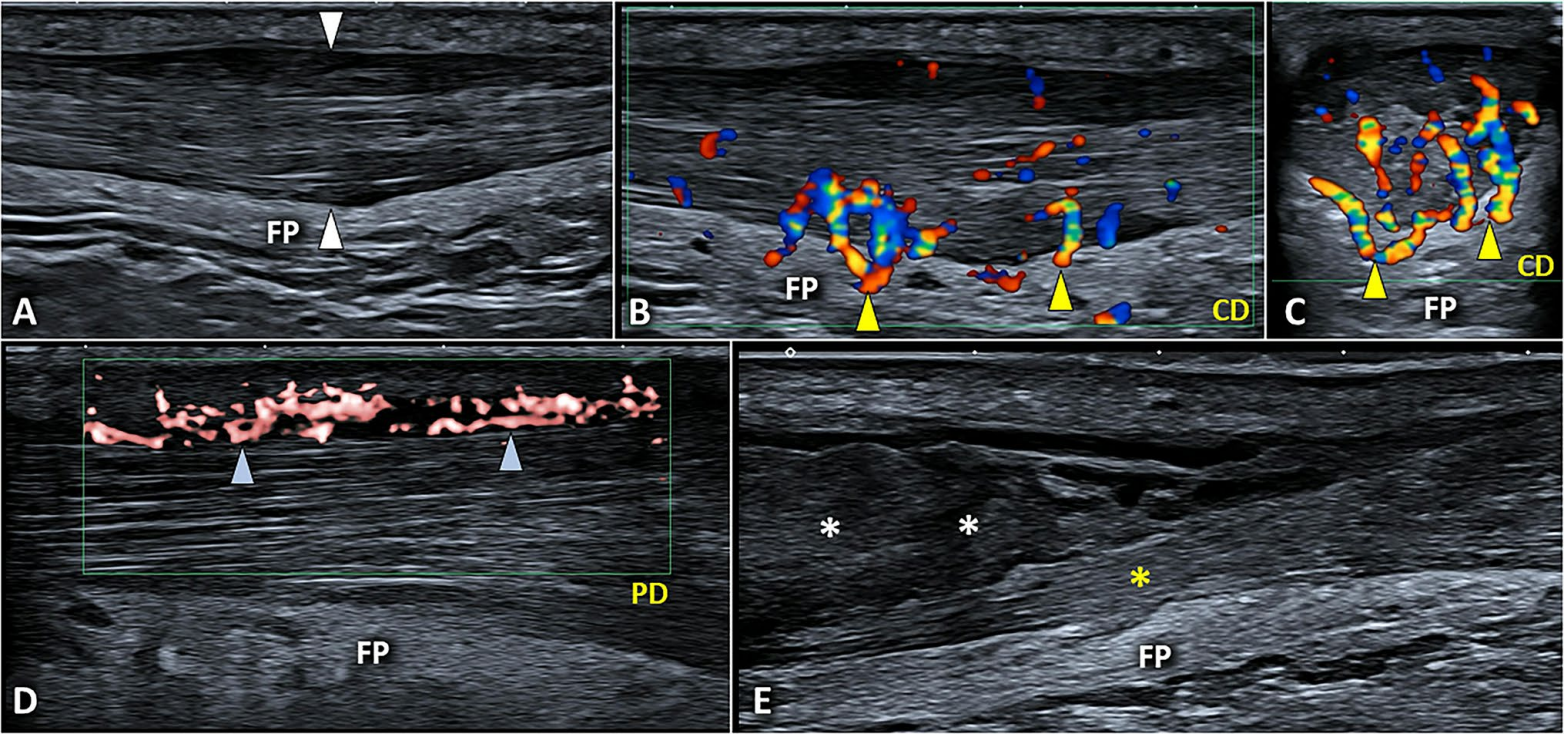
Fusiform hypoechoic thickening *(white arrowheads)* of the midportion of AT and its hypervascularization with neo-vessels *(yellow arrowheads)* originating from the underlying Kager fat pad *(FP)* can be considered the most common sonographic signs of non-insertional pathology (A–C). More rarely, isolated peri tendinitis may be identified with vascular signals *(blue arrowheads)* confined inside the thickened paratenon and a normal thickness/echotexture of the underlying AT (D). In players with suspected partial injury of the AT, ankle dorsiflexion may be performed to dynamically and selectively glide the disrupted tendon fibers *(white asterisks)* confirming the diagnosis (E). *CD* color doppler, *PD* power doppler
